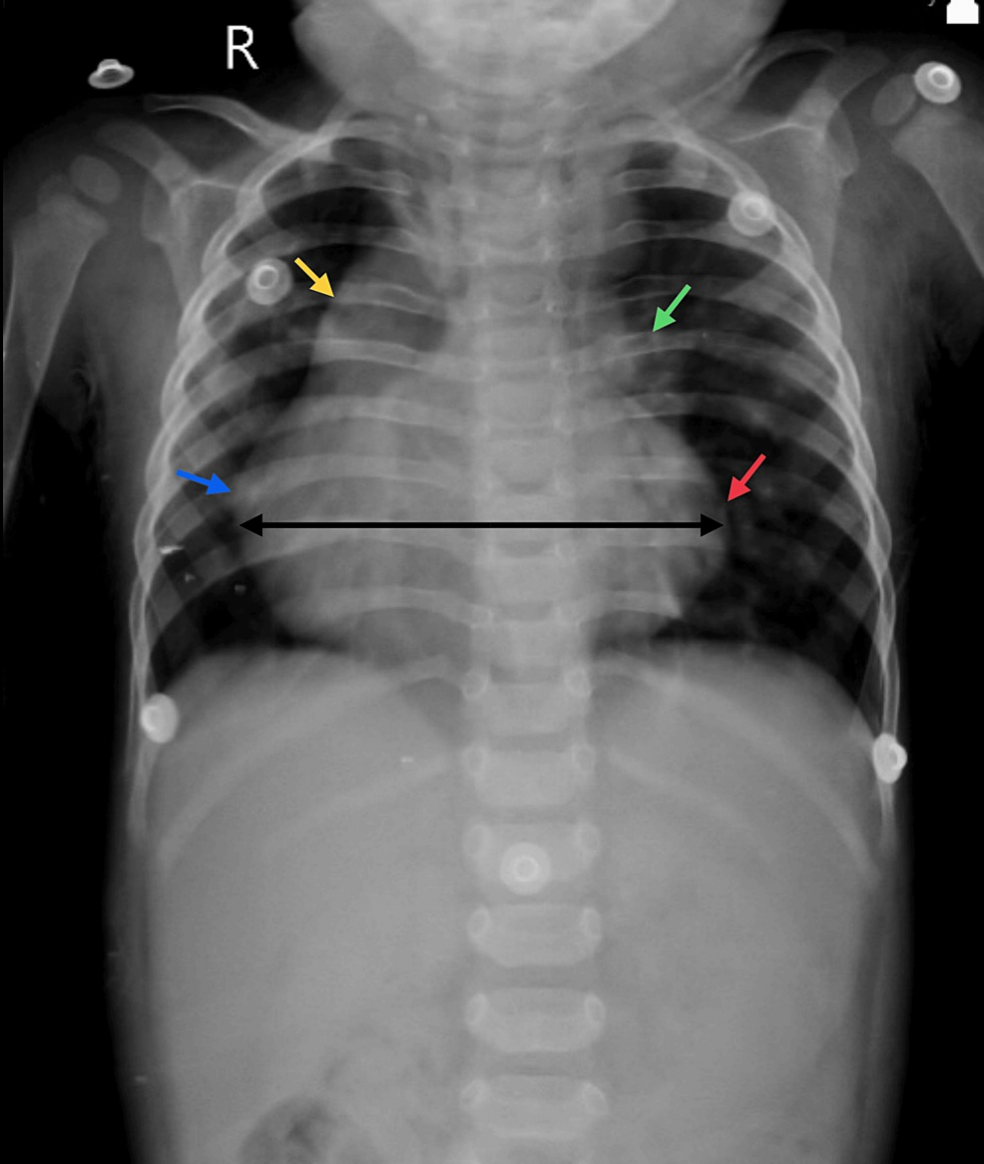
Chest X-ray showing RA enlargement (marked by the yellow arrow), RV enlargement (marked by the blue arrow), RV apex (marked by the red arrow), and hump suggestive of AOLPA (marked by the green arrow) The image was taken by the authors. R: right atrium, RV: right ventricle, AOLPA: anomalous origin of left pulmonary artery
